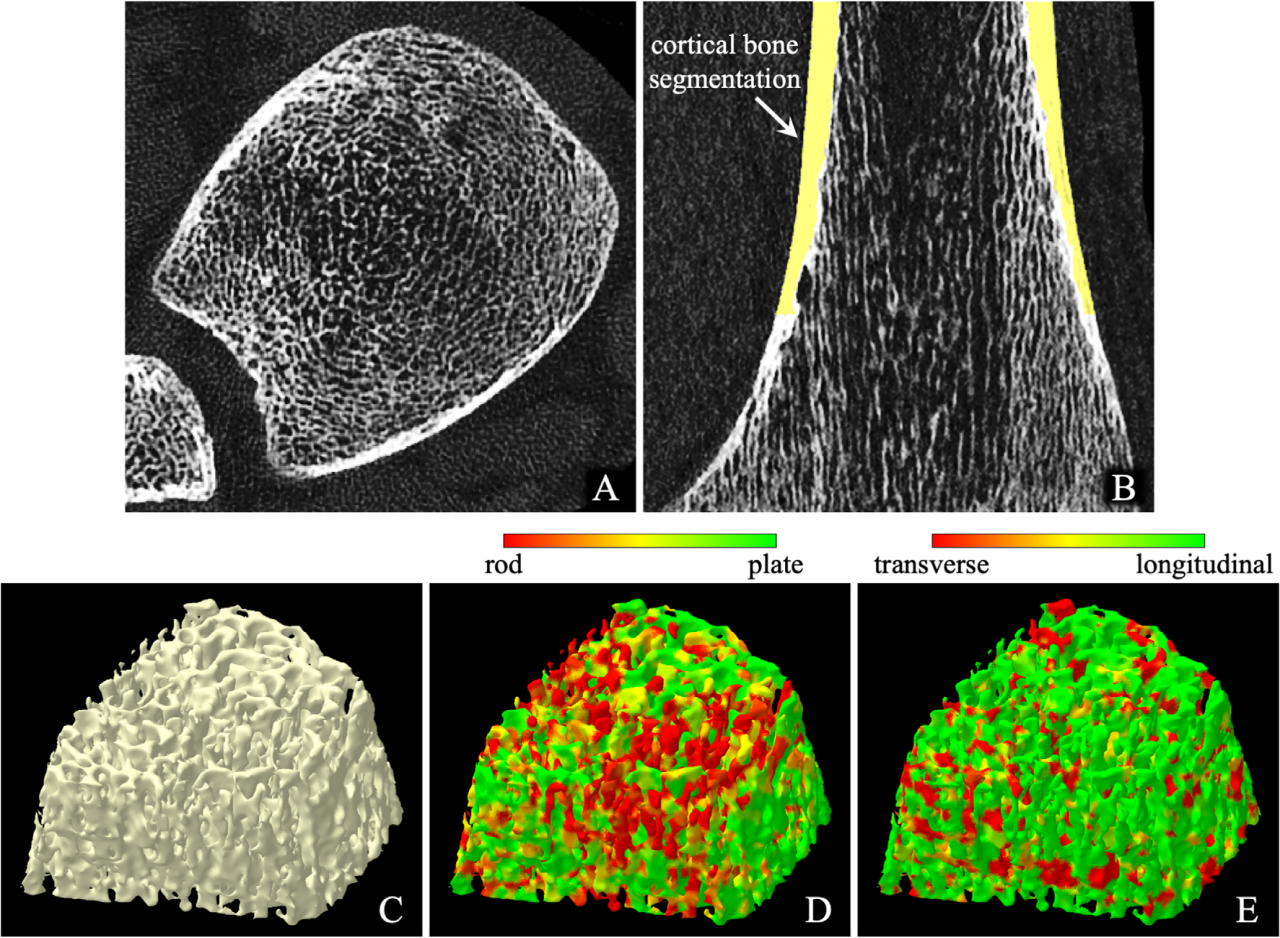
In vivo imaging of bone microstructure on a Siemens SOMMATOM Force CT scanner. (*A*, *B*) Axial and coronal image slices from a distal tibia CT scan. Cortical bone (Cb) segmentation over 8% to 16% of distal tibia is overlaid in (*B*). (*C*) Surface rendition of the Tb network at the inner region with 60% peel from the outer Cb surface. (*D*, *E*) Color‐coded displays of trabecular plate‐rod (*D*) and transverse‐longitudinal (*E*) microstructure.
